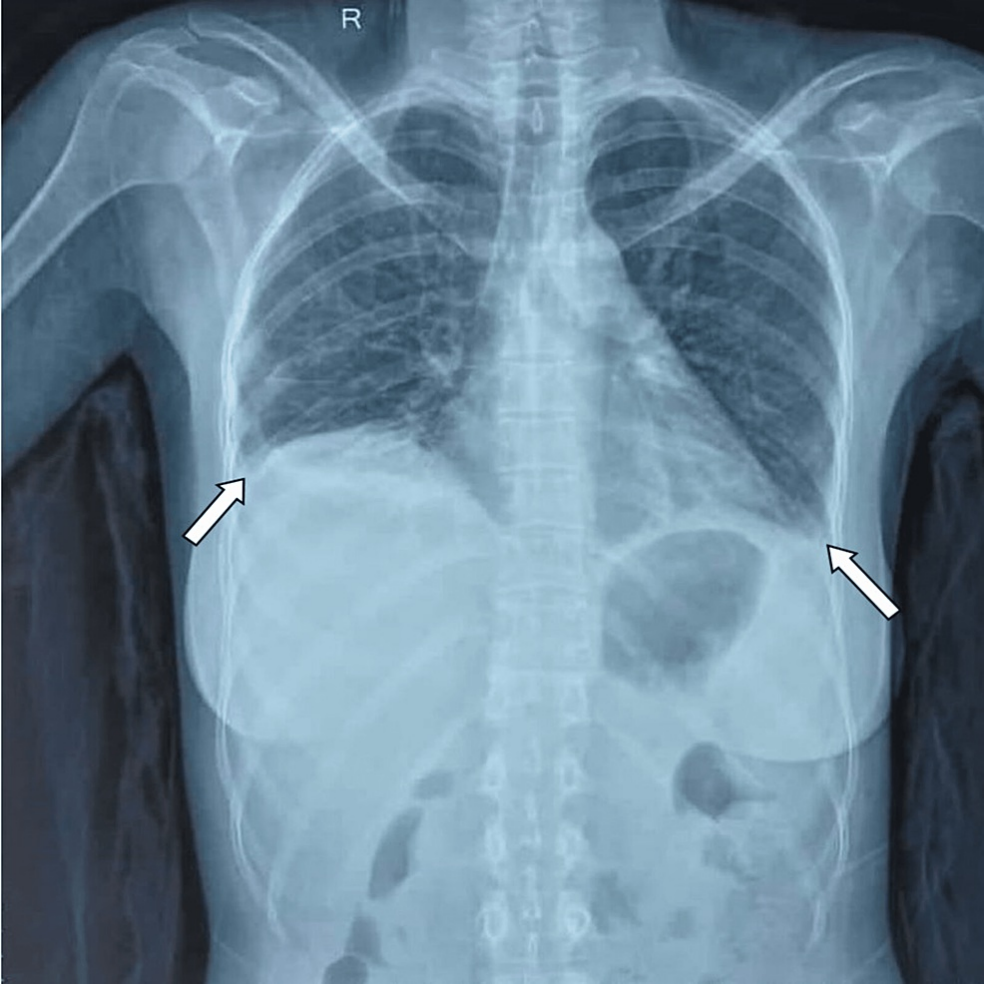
Chest x-ray suggestive of bilateral pleural effusion (white arrow).
